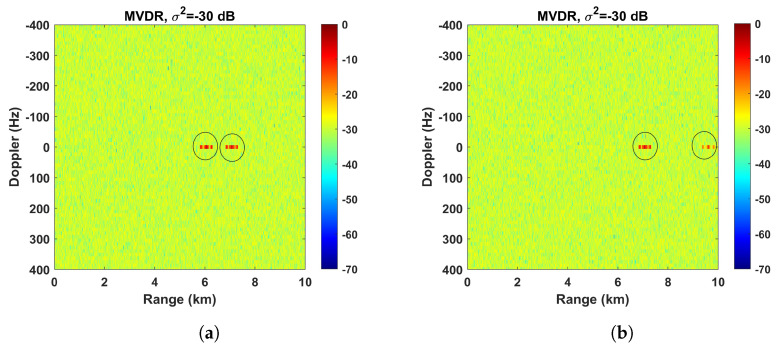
Range–Doppler map applying the MVDR beamforming technique after the CAF where the interference signals are not removed correctly: (a) target #1 is not removed; (b) target #3 is not removed.
